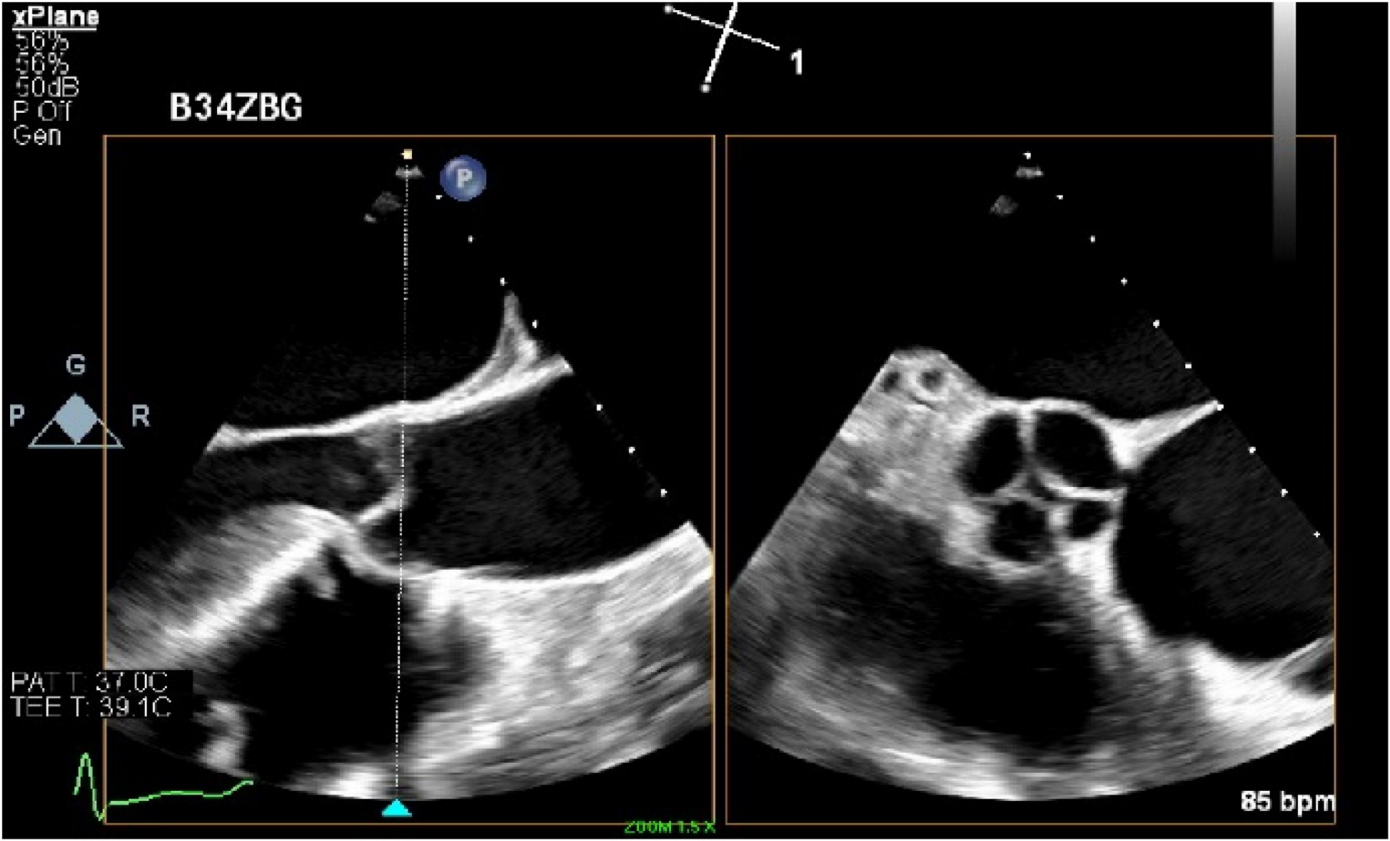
Image of the quadricuspid aortic valve captured via transesophageal echocardiography (TEE) in bi-plane view, highlighting the rare four-cusp morphology.
